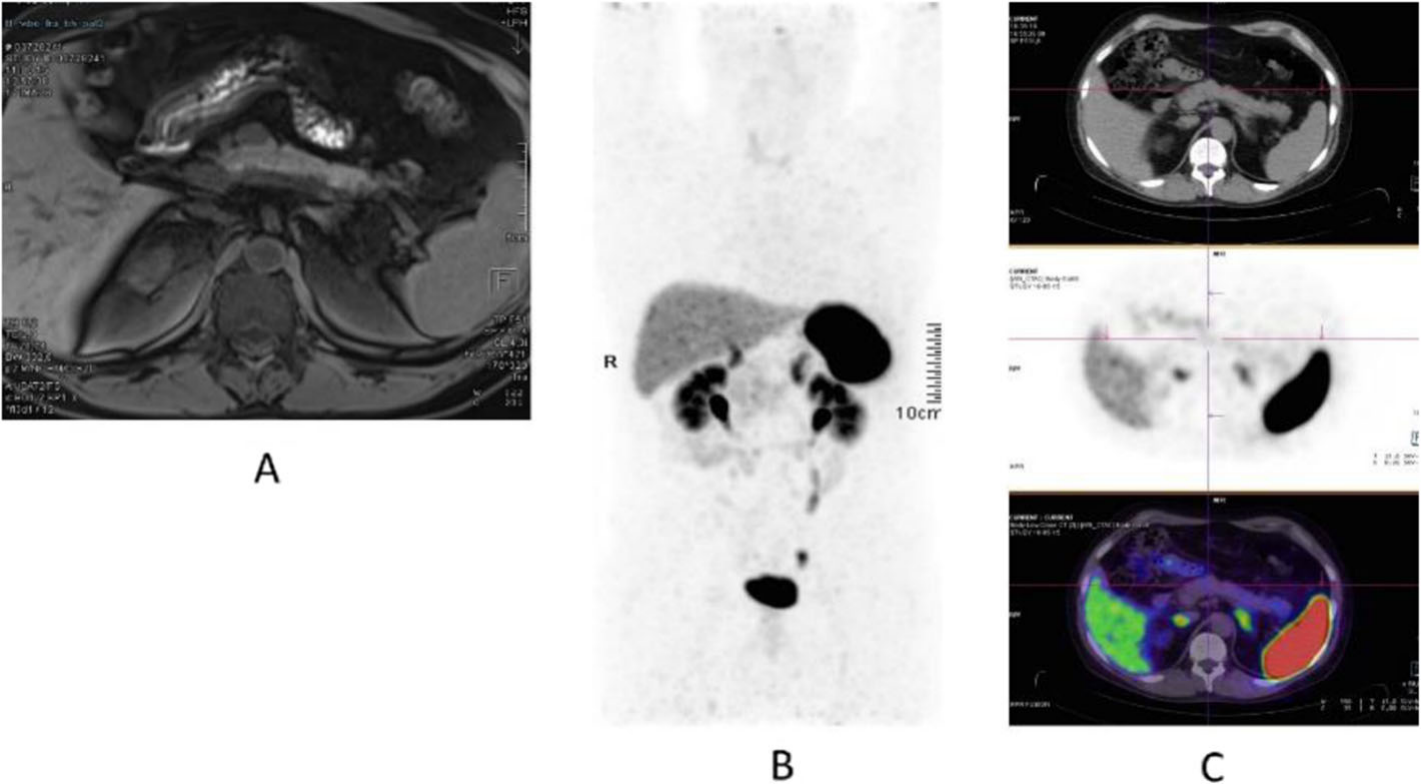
^68^Ga-DOTA peptide, suspected lesion of the pancreas, false negative. *Clinical history*: 48 y.o. man with lesion in the pancreatic body (a MRI). Clinical suspicion of insulinoma (hypoglycaemias). *PET/CT findings*: no uptake in the lesion (b MIP, c CT, PET, and fused images). The patient underwent surgery, which confirmed the presence of an insulinoma (frequently false negative with ^68^Ga-DOTA peptide)
